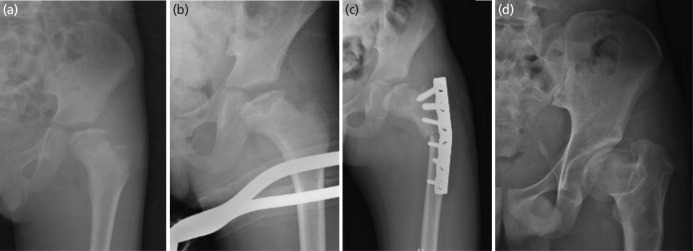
The series of radiographs belongs to patient no.6. (a) The presentation with Herring C and pseudo hinge abduction at age 11 years old. (b) The hip reduced with traction for 10 days. (c) The immediate post-surgery showed good containment. (d) The hip at maturity showed relatively greater trochanteric overgrowth, coxa magna, coxa breva and classified as Stulberg III.
